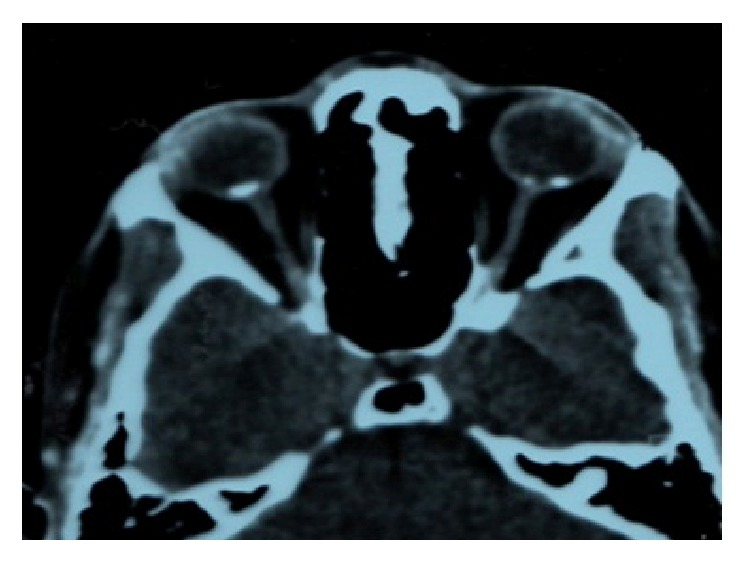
Orbital computerized tomography (CT) scans show hyperdense plaques, with bone-like homogeneity in the posterior pole. Two plaques are seen in the left eye.
